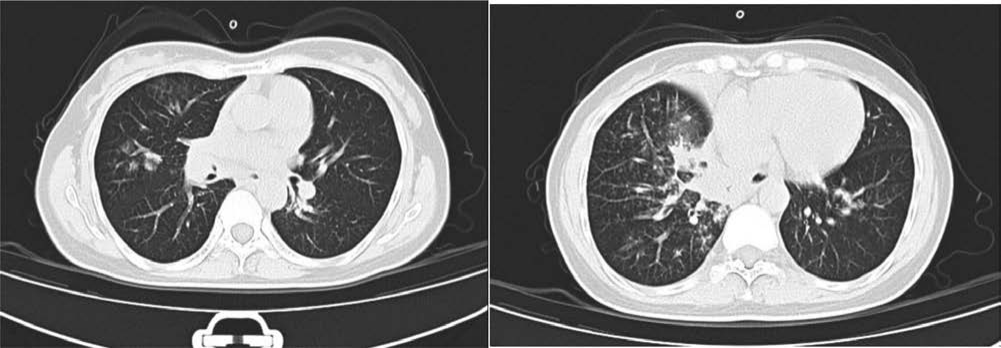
Chest CT showed bilateral multiple patchy high-density shadows, multiple bronchial wall thickening in both lungs, partial lumen stenosis, and local lamellar consolidation in the lower lobe of the right lung. Multiple lymph nodes are seen in the mediastinum; multiple infections in both lungs, local atelectasis in the right lung, multiple bronchial mucus plugs in both lungs. Multiple mediastinal lymph nodes.
